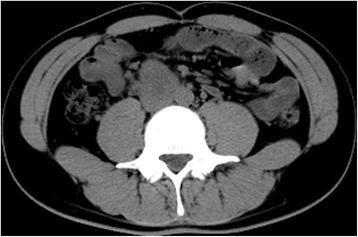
Computed tomographic scan displaying the suspicious metastasis sites in the retroperitoneal lymph nodes (the soft tissue density shadows anterior to the right psoas and at the level of the fourth lumbar vertebra)
